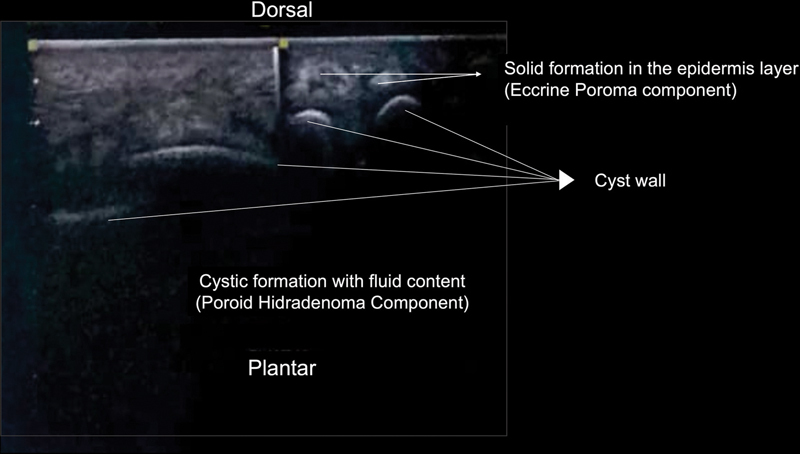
Ultrasound image showing a well-defined translucent cystic formation with a homogeneous fluid content within the dermis layer (PH component) and a solid formation in the epidermis layer (EP component).
